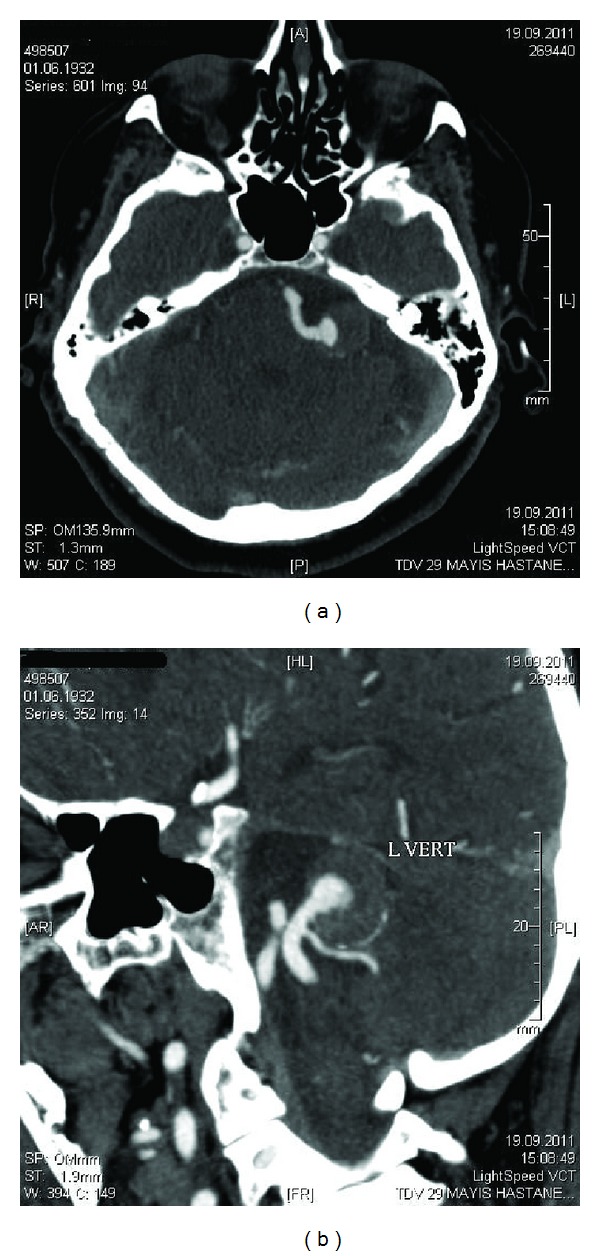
(a) and (b) CT angiography shows partially thrombosed saccular aneurysm with calcified walls, emerging from left PICA, just distal to its origin from vertebral artery on axial and saggital plane.
